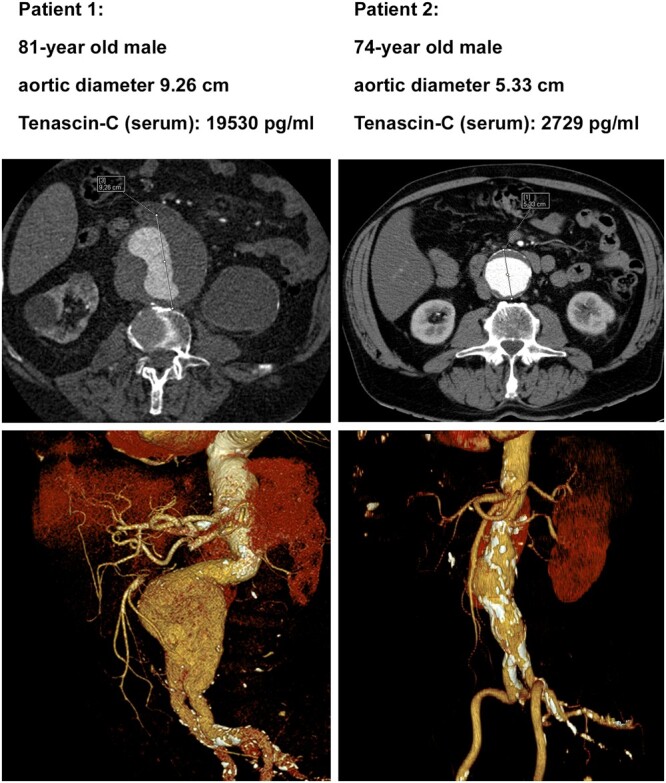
Representative three-dimensional reconstructed computed tomography images as well as tenascin C serum levels obtained from 2 abdominal aortic aneurysms patients.
